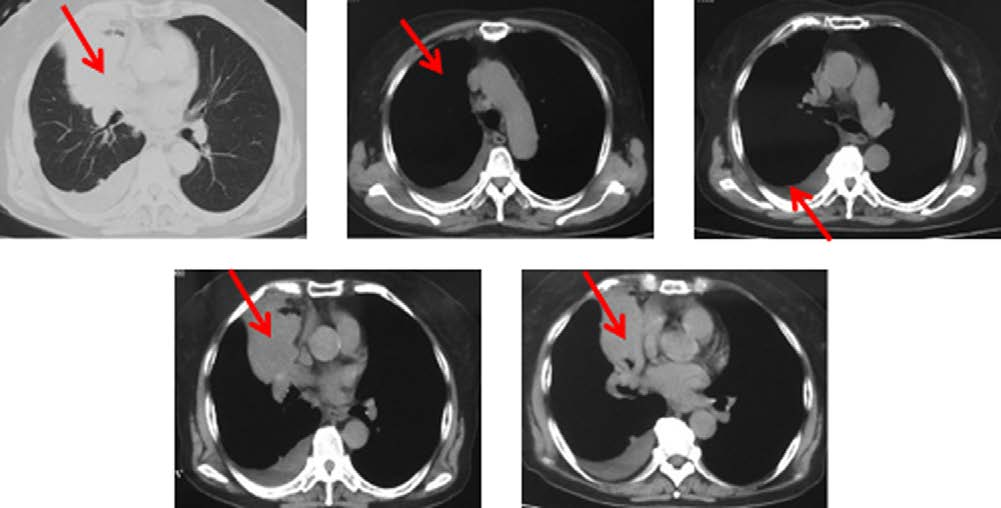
The chest CT scan demonstrated right middle lobe mass, right hilum and mediastinal lymph node metastasis, right pleural effusion at baseline. CT=computed tomography.
